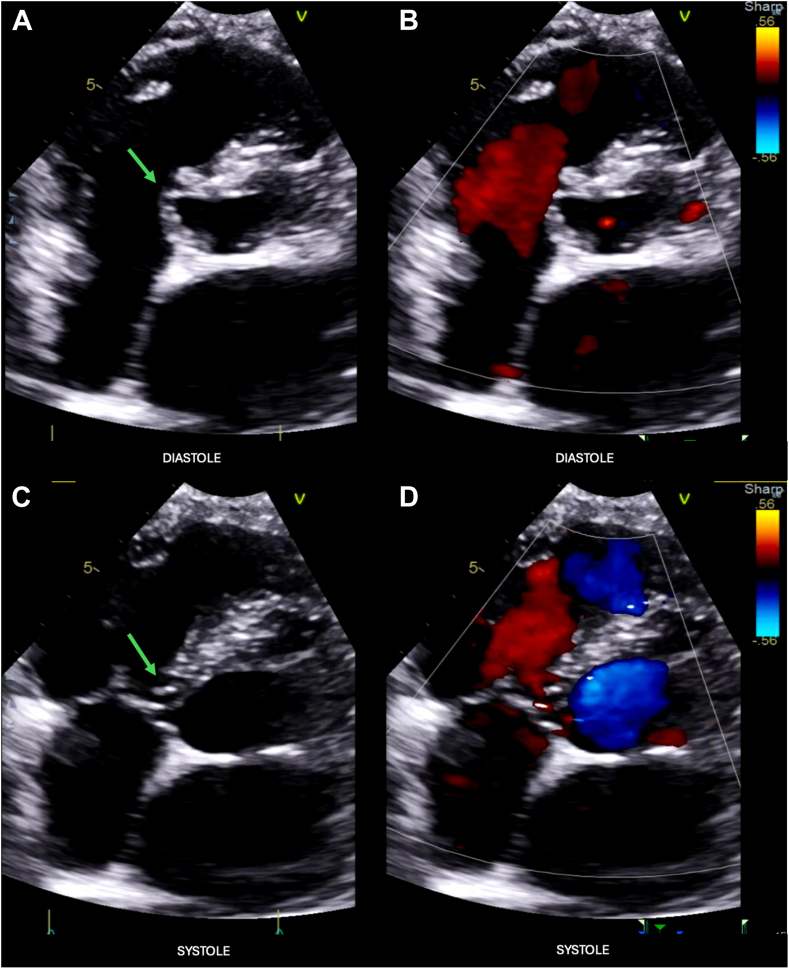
Two-dimensional TTE without (*left*) and with (*right*) color-flow Doppler, basal parasternal long-axis diastolic (A) and B and systolic (C and D) views, demonstrates a narrow-necked saccular outpouching anatomic defect that arises from the membranous septum, just beneath the aortic annulus, extends into the right ventricle adjacent to the septal tricuspid leaflet, is noncontracting, and is without evidence of left-to-right shunt, consistent with an IVMSA (*arrows*).
